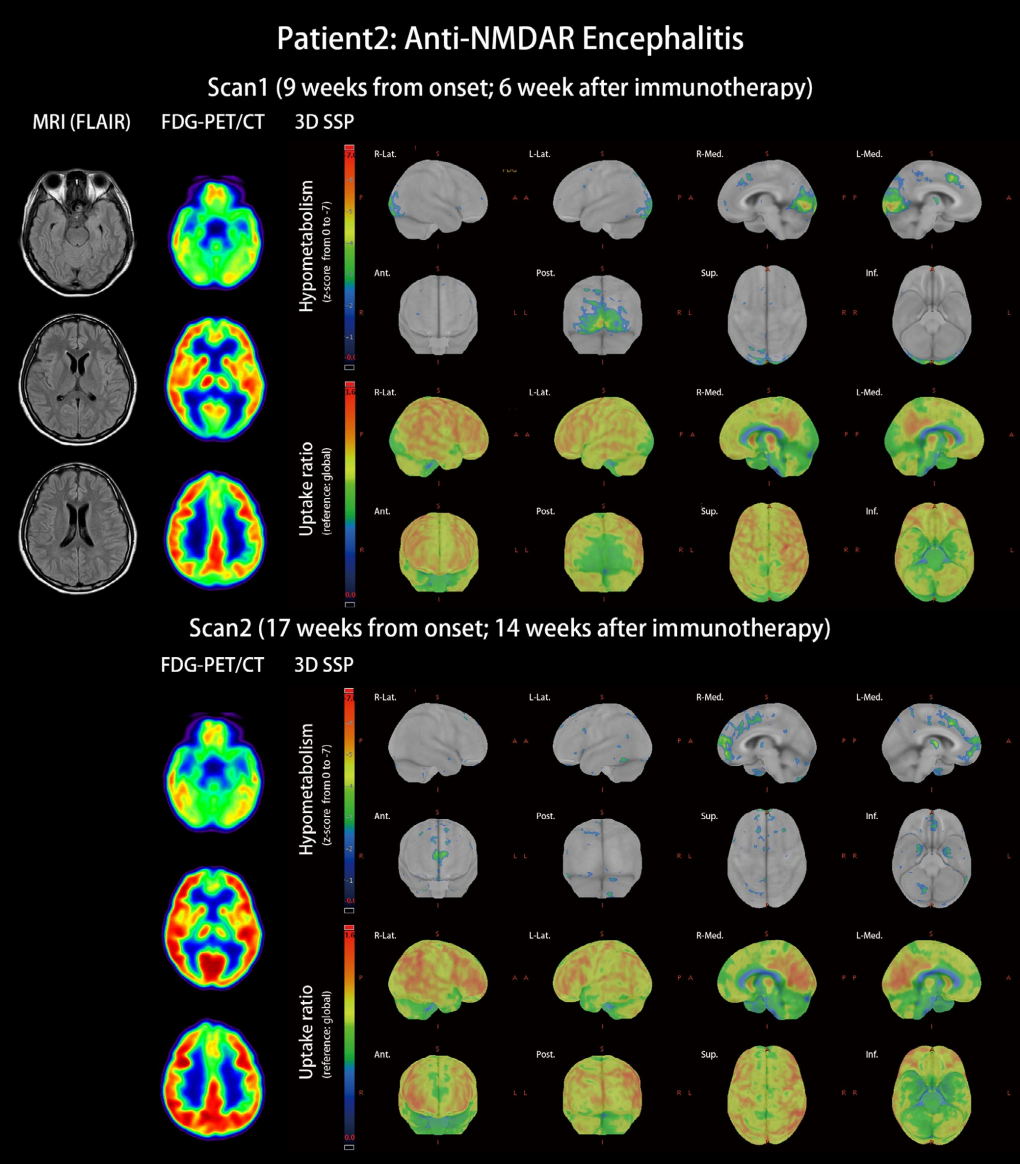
MRI and FDG‐PET/CT of anti‐NMDAR encephalitis (patient 2). A 29‐year‐old female patient with subacute psychosis, consciousness alternation, and status epilepticus for 3 weeks was diagnosed as having anti‐NMDAR encephalitis. T2‐weighted FLAIR images of MRI revealed mild edema and hyperintensities of the bilateral mesial temporal area. After 6 weeks of immunotherapy, FDG‐PET/CT at the 9th week from the first symptoms revealed occipital‐dominant hypometabolism, which was compatible with previously reported distinct hypometabolic patterns of anti‐NMDAR encephalitis. Relative hypermetabolism at the primary sensorimotor cortices, lateral temporal area, inferior parietal area, and pons was noticed in the uptake ratio of scan 1. The occipital hypometabolism resolved in the second PET scan at the 17th week from onset, but new medial frontal to anterior cingulate hypometabolism occurred
